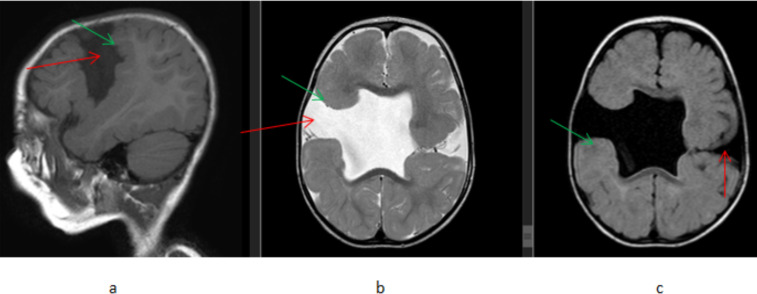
A 1-year-old male patient presented with developmental delay and seizure, (a) T1W sagittal (b) T2W axial and (b) FLAIR axial, brain MR images showing bilateral posterior frontal lobe CSF filled clefts(red arrows) which are grey matter lined(green arrows) that extend from surface to the body of lateral ventricles. There is also an absent septum pellucidum. Findings are consistent with bilateral Schizencephaly
